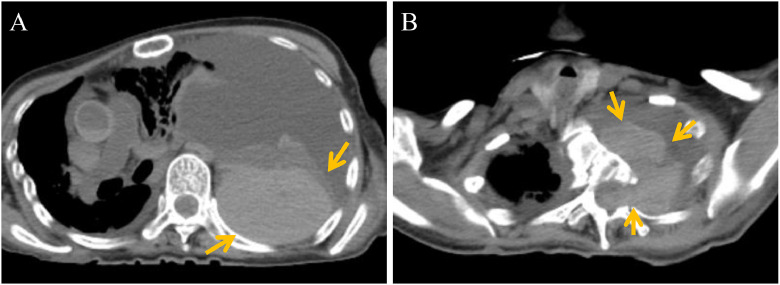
Postmortem CT. Postmortem CT revealed intrathoracic fluid accumulation and high-attenuation hematoma predominantly located in the dorsal aspect of the left thoracic cavity (A, arrow). The meningocele in the left thoracic cavity was also filled with high-attenuation hematoma (B, arrow), suggesting the presence of antemortem hemorrhage. Mediastinal shift to the right was observed due to the left-sided hemothorax.
